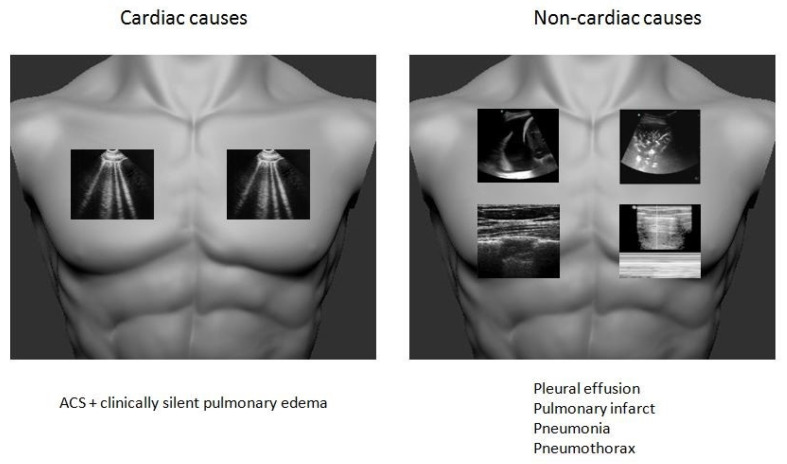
Lung sonographic decision making algorithm in chest pain. ACS: acute coronary syndrome.
